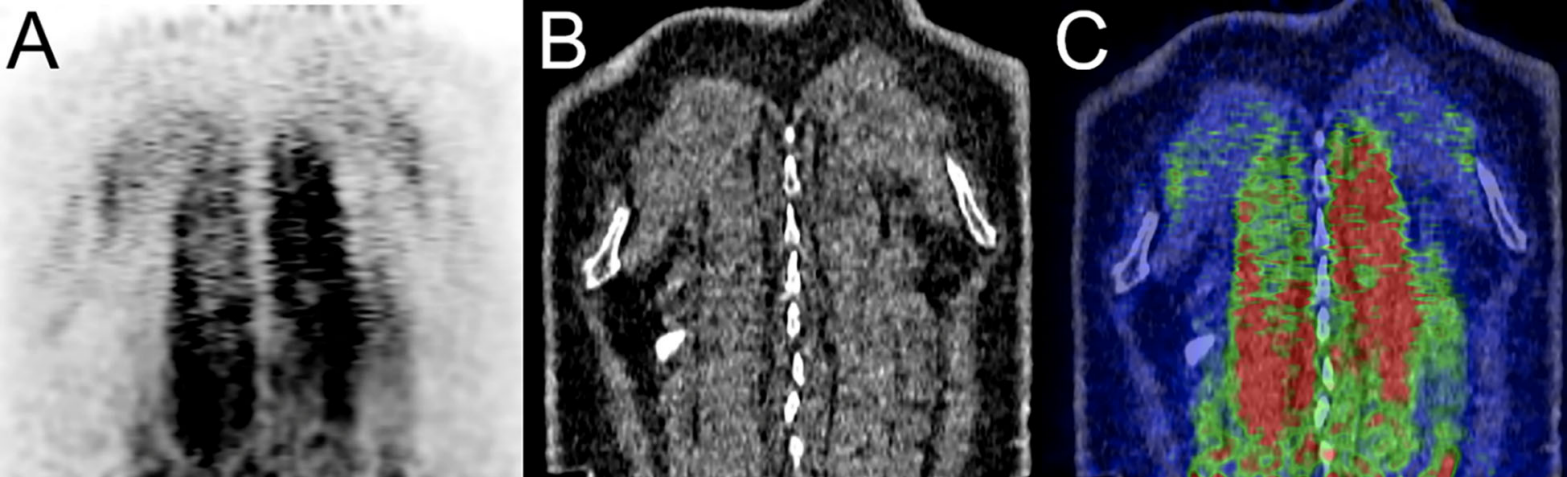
Immune-related myositis in a 61-year-old male patient with small cell neuroendocrine carcinoma of the right lung hilum (cT4 cN2 cM0, stage IIIB) initially treated with chemo-radiation therapy who developed diffuse metastatic disease. A treatment with ipilimumab (anti-CTLA-4) and nivolumab (anti-PD-1) was administered. At 1-month post-immunotherapy, a ^68^Ga-DOTATOC-PET/CT showed diffuse myositis of paraspinal muscles coronal PET, CT and fused PET/CT images (A–C) respectively showing spinalis, longissimus thoracis and iliocostalis thoracis muscles.
